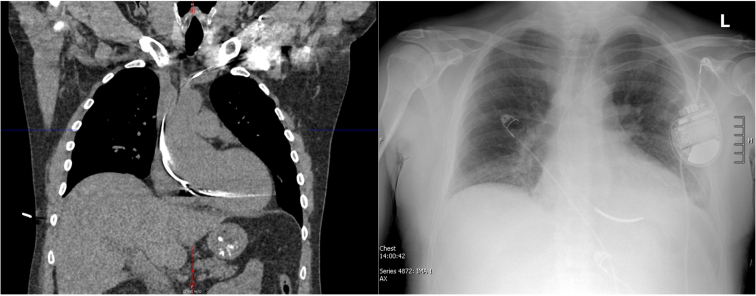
Preimplant computed tomography scan (*left*) and radiograph of the chest (*right*).
